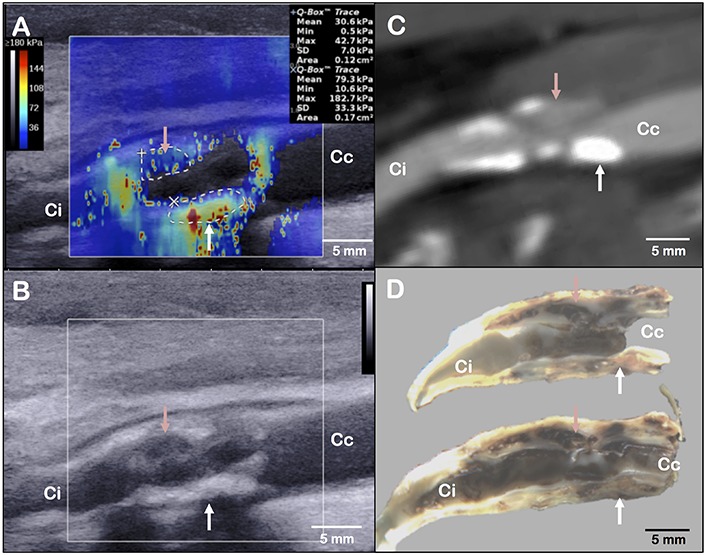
Layout of elastography assessment (A), B-mode ultrasound acquisition (B), computed tomography angiography (C) and gross examination (D) of the same plaque in a longitudinal axis view. The red arrow indicates the main haemorrhagic area on panel (D). It corresponds to a low stiffness (A), hypoechogenic (B) and low-density (C) area. The white arrow indicates a fibrous and calcified area on panel (D). It corresponds to a high stiffness (A), hyperechogenic (B) and high-density (C) area. Ci, internal carotid; Cc, common carotid.
